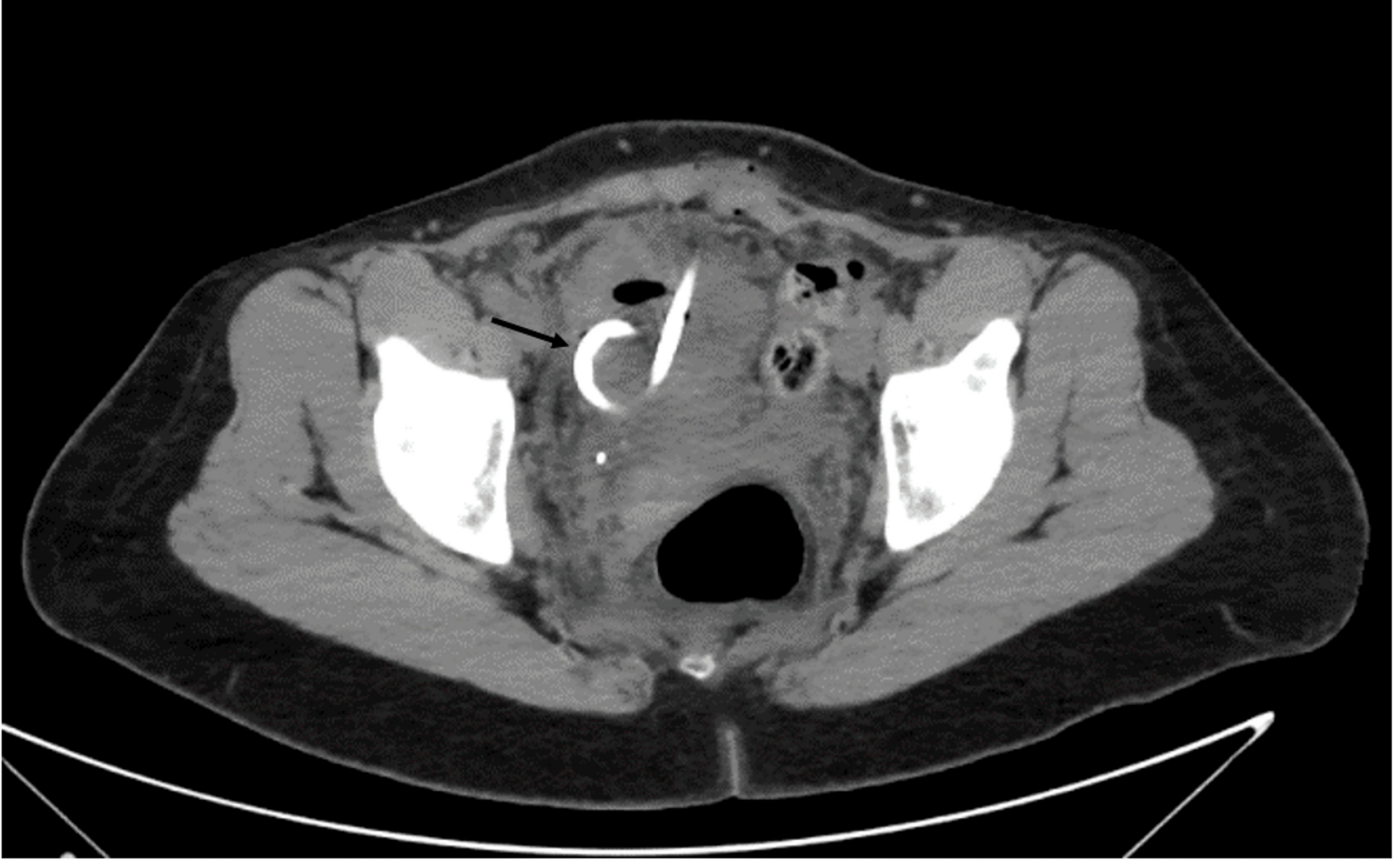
CT image during pigtail catheter placement CT axial images of the pelvis during pigtail catheter placement (black arrow) shows that the  fluid-like collection collapsed.
